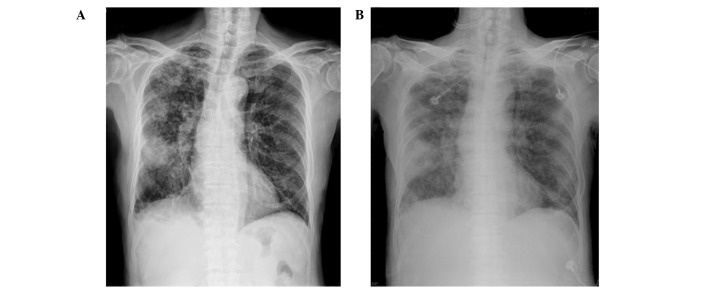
Chest radiograph shows an ill-defined mass lesion ∼5 cm in size at the (A) right middle lung zone at first admission and (B) diffuse infiltration and ground glass opacities over bilateral lung fields outside the mass at readmission.
